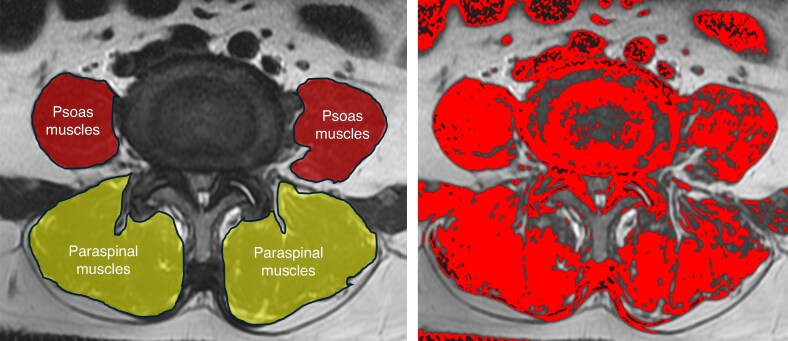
Bilateral manual segmentation of psoas and posterior paraspinal muscles on preoperative axial T2-weighted magnetic resonance imaging Cross-sectional area measured by Image J.
